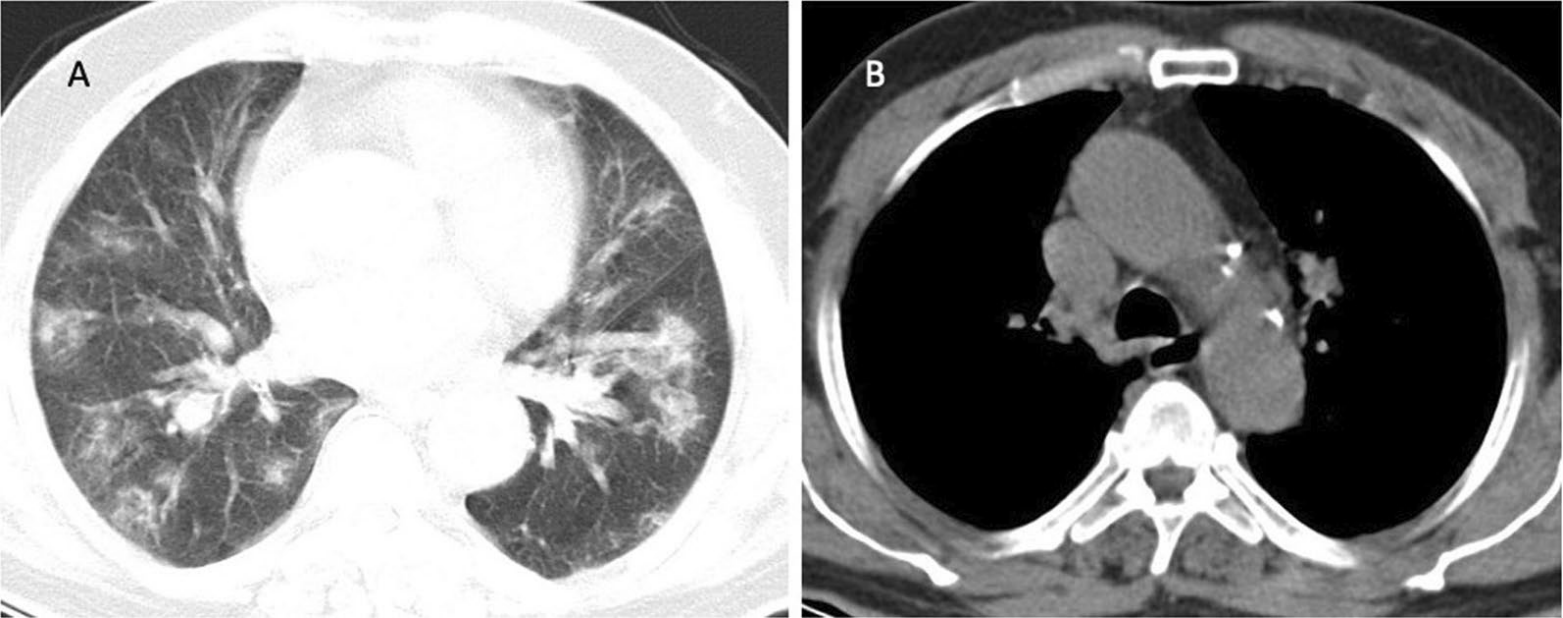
66-year-old male COVID-19 (+) patient with CT-SS 20 (A) and fatty thymus (B)
